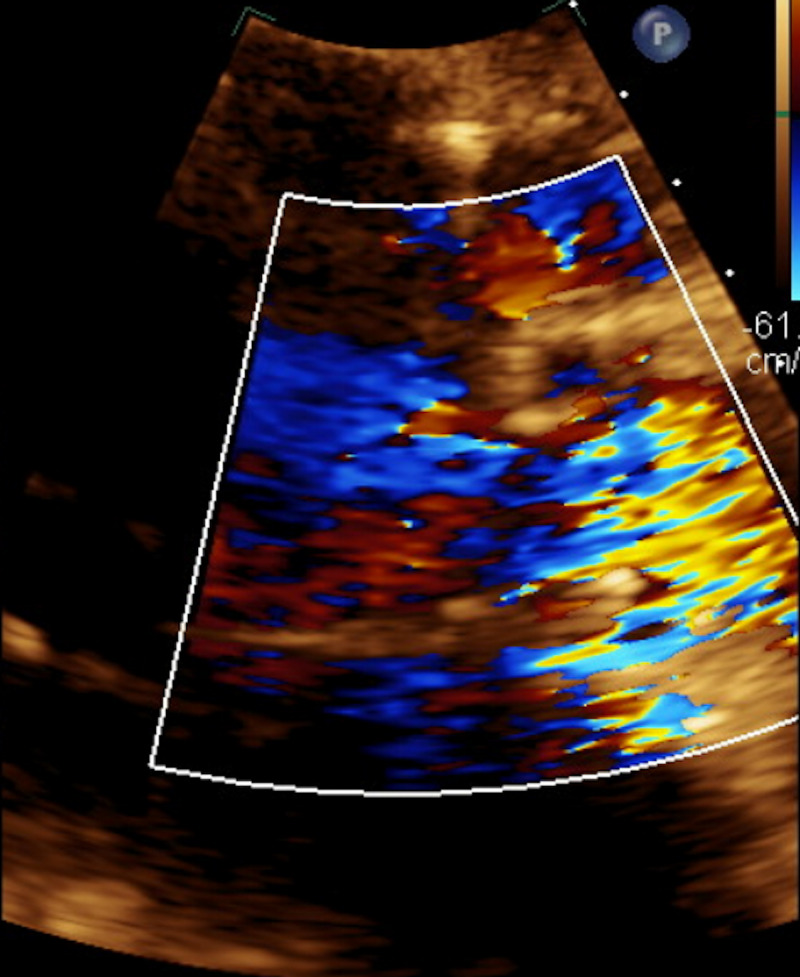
Severe Aortic Regurgitation The multispectral color doppler image demonstrates retrograde flow from the ascending aorta (in blue) consistent with aortic regurgitation.
